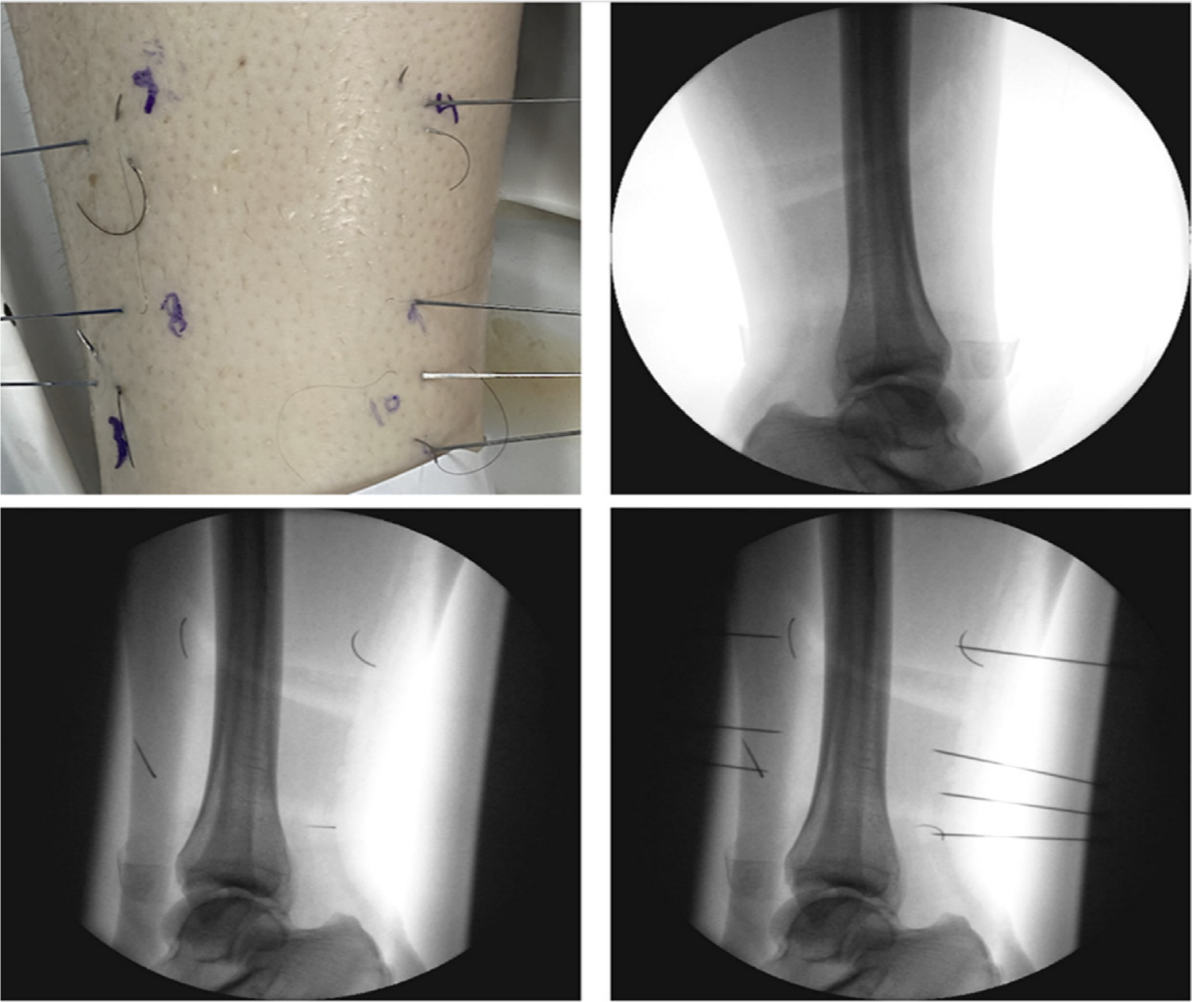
Distal tibia images/radiographs for needle identification.
